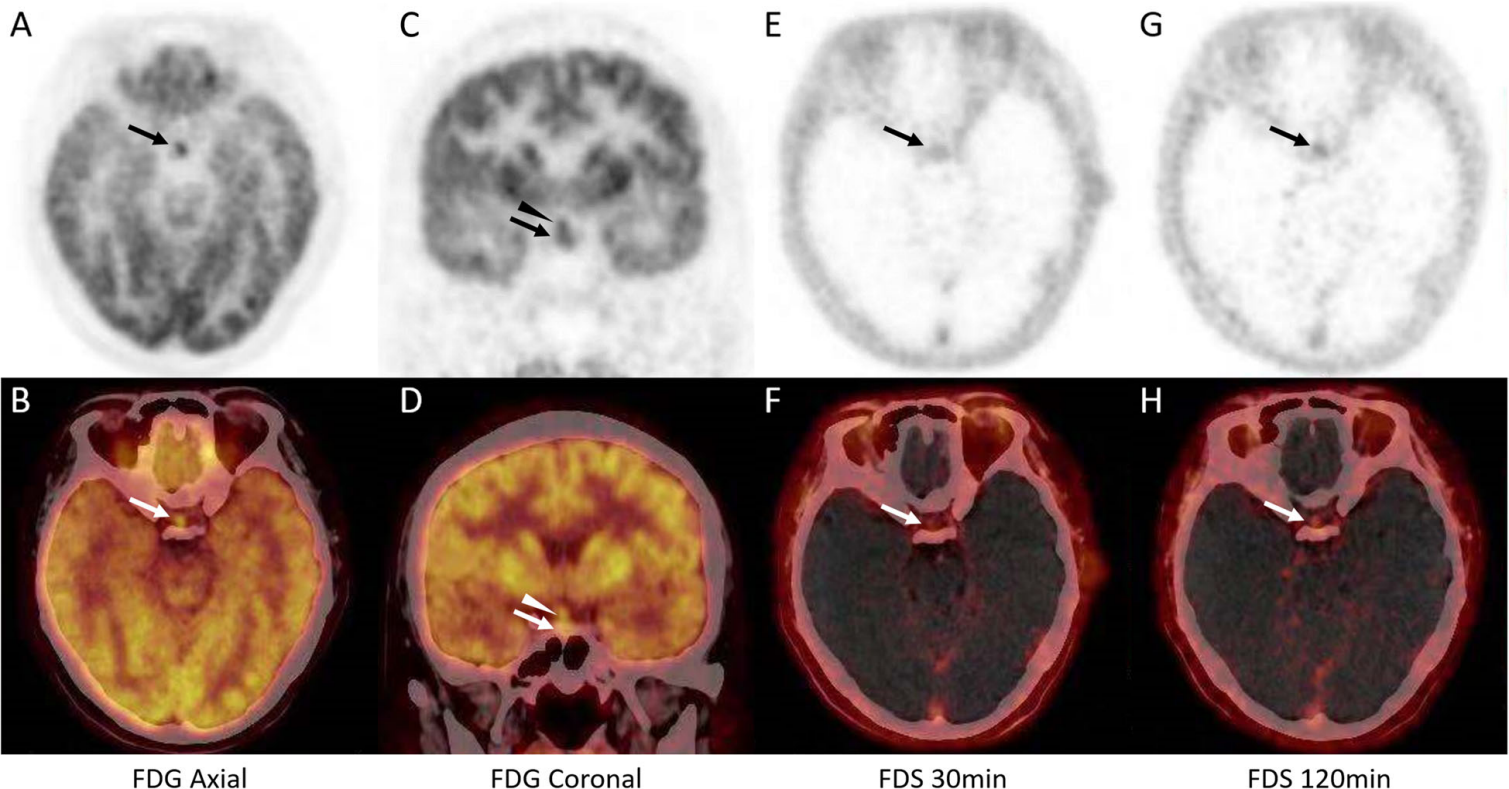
FDG and FDS activity of the lesions. a-d. Both the pituitary and pituitary stalk lesions displayed FDG activity with an SUVmax of 5.5 after 40 min of radiotracer injection. e-f. Both lesions displayed FDS activity with a SUVmax of 1.1 at 30 min after FDS injection; in comparison, the SUVmax of the normal brain tissue was 0.15, and the T/N ratio was 7.3. g-h. The lesions remained FDS active after 120 min of FDS administration with a SUVmax of 0.73; the SUVmax of the normal brain and the T/N ratio were 0.08 and 9.1, respectively
